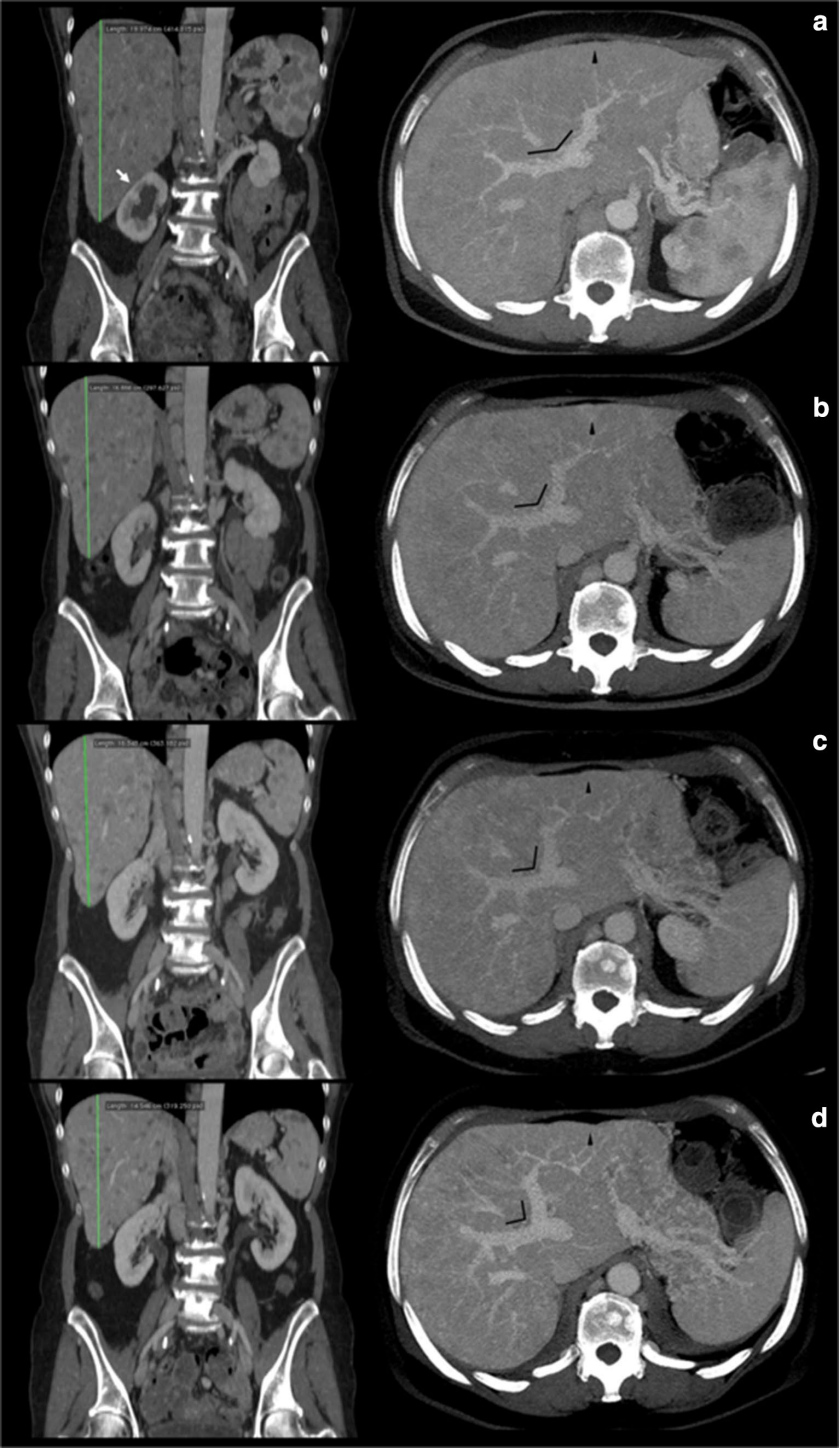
Hepatomegaly reduction. CT portal-phase contrast-enhanced images: Coronal plane reconstruction and Axial Maximum Intensity Projection (MIP) reconstruction at time 0 (a) and at 4 (b), 7 (c), 10 month (d) follow up examinations. Progressive reduction of longitudinal diameter of the liver measured in the mid-clavicular line was observed at follow up study. Progressive reduction of lower margin extension below costal arch was also detected. Caudal displacement of right kidney at time 0 (*white arrow*) gradually disappears at successive studies. Axial MIP images show progressive reduction of the displacement effect on the portal bifurcation due to decrease of metastatic parenchymal lesions: space between left and right portal trunk (*angle*) gradually decrease over time. Note the increased caliber of portal trunks and progressive reduction of the swelling of liver profile (*arrowhead*) related with hepatomegaly, with increased thickness of perivisceral fat
